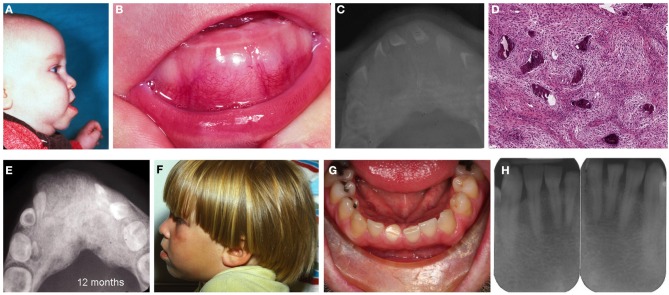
Patient II-2. (A) Profile of the patient exhibiting enlargement and protrusion of the mandible, (B) lobular enlargement of the mandible, (C) occlusal radiograph depicting enlargement of the middle aspect of the mandible characterized by a ground-glass pattern, (D) loose cellular fibroblastic proliferation with deposits of woven bone, (E) radiograph revealing irregular ground glass-like appearance of the mandible and splaying of teeth. (F) profile of the patient at age 3 year, (G) patient as an adult at age 33 years. No evidence of jaw enlargement was present, (H) no evidence of ground glass appearance or enlargement.
